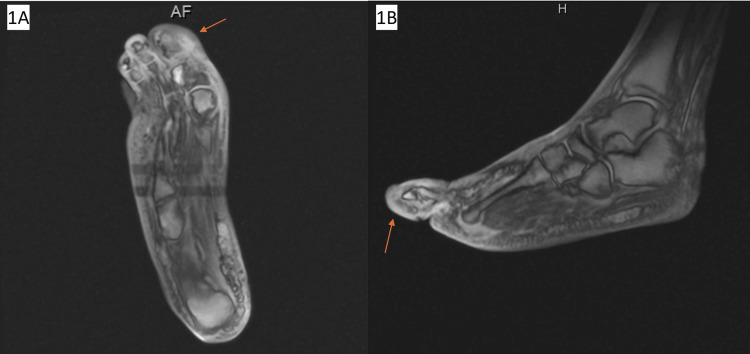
MRI of the right lower extremity in the axial plane (A) and sagittal plane (B) showing diffuse subcutaneous edema consistent with cellulitis (red arrow), but no signs of osteomyelitis.
